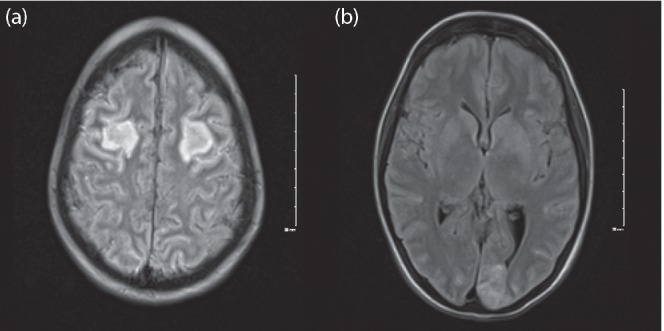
(a) MRI axial T2 FLAIR image demonstrating bilateral frontal acute infarction (b) MRI axial demonstrating acute left occipital and subactue caudate stroke.
